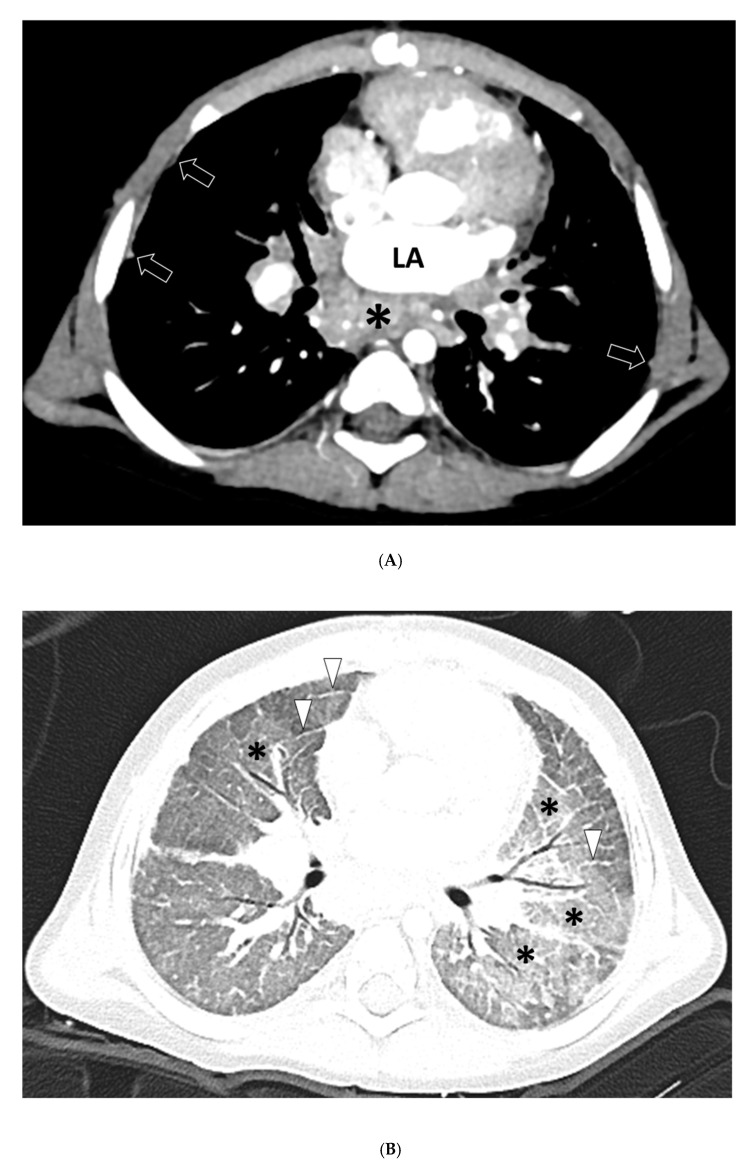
A 4-year-old male with complete atrioventricular canal defect and pulmonary vein stenosis who presented with pulmonary hypertension. (A) Axial contrast-enhanced thoracic MDCT angiography CT image shows ill-defined, mildly heterogeneously enhancing, non-calcified soft tissue mass (asterisk) in the expected location of bilateral pulmonary veins. Pleural thickening (arrow) is also seen. LA = Left atrium. (B) Axial lung window CT image demonstrates ground-glass opacities (asterisks) in both lungs and septal thickening (arrowheads).
